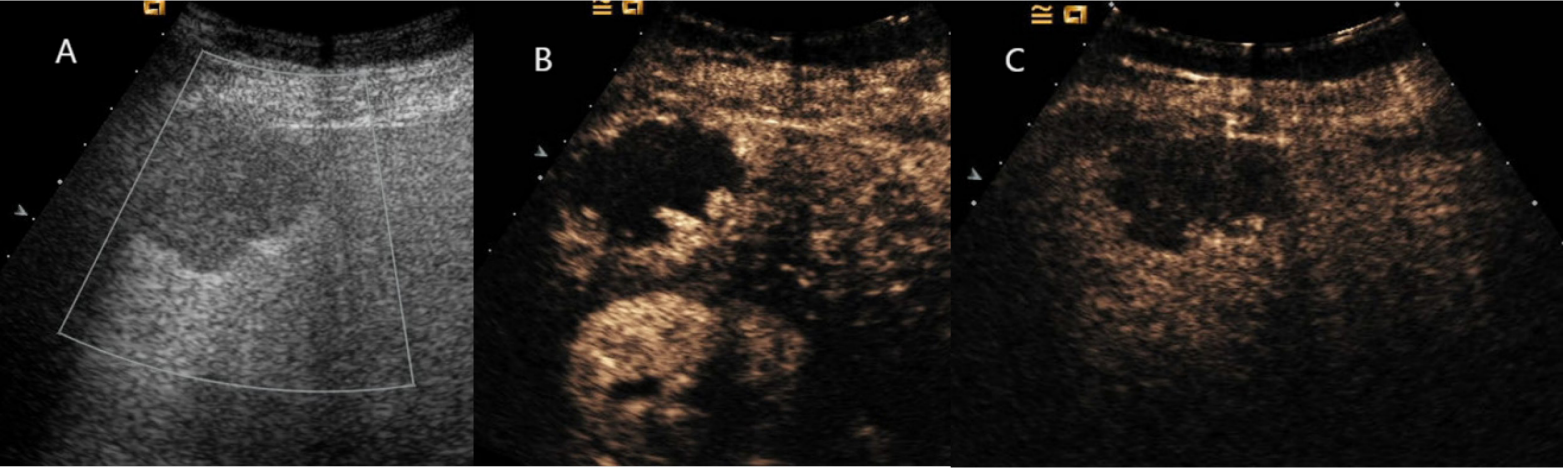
Hepatic hemangioma showed peripheral nodular enhancement in all vascular phases (A) Ultrasound revealed a hypoechoic mass under right liver capsular. (B) and (C) CEUS showed peripheral nodular enhancement throughout the whole vascular period.
